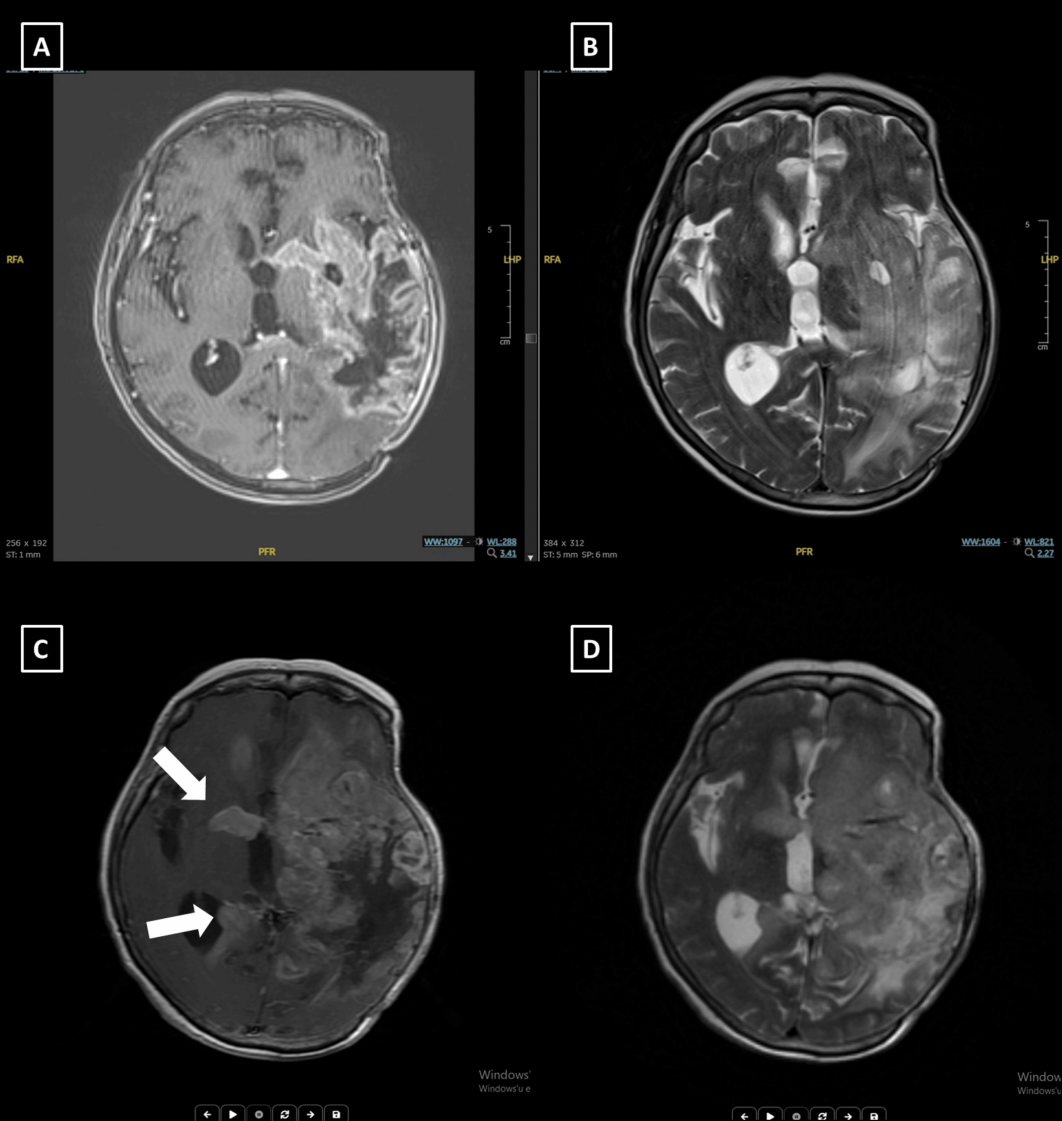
Diagnostic and 3-month follow-up MRI images of Case 4. (A, B) Diagnostic MRI: Axial T1-weighted post-contrast (T1C+) (A) and T2 fluid-attenuated inversion recovery (FLAIR) (B) images showing a recurrent lesion involving the left anterior temporal lobe, basal ganglia, and posterior insular region. (C, D) Three-month follow-up MRI after LATTİCE radiotherapy: Axial T1C+ (C) and T2 FLAIR (D) images demonstrate lesion progression with extension into the contralateral hemisphere.
